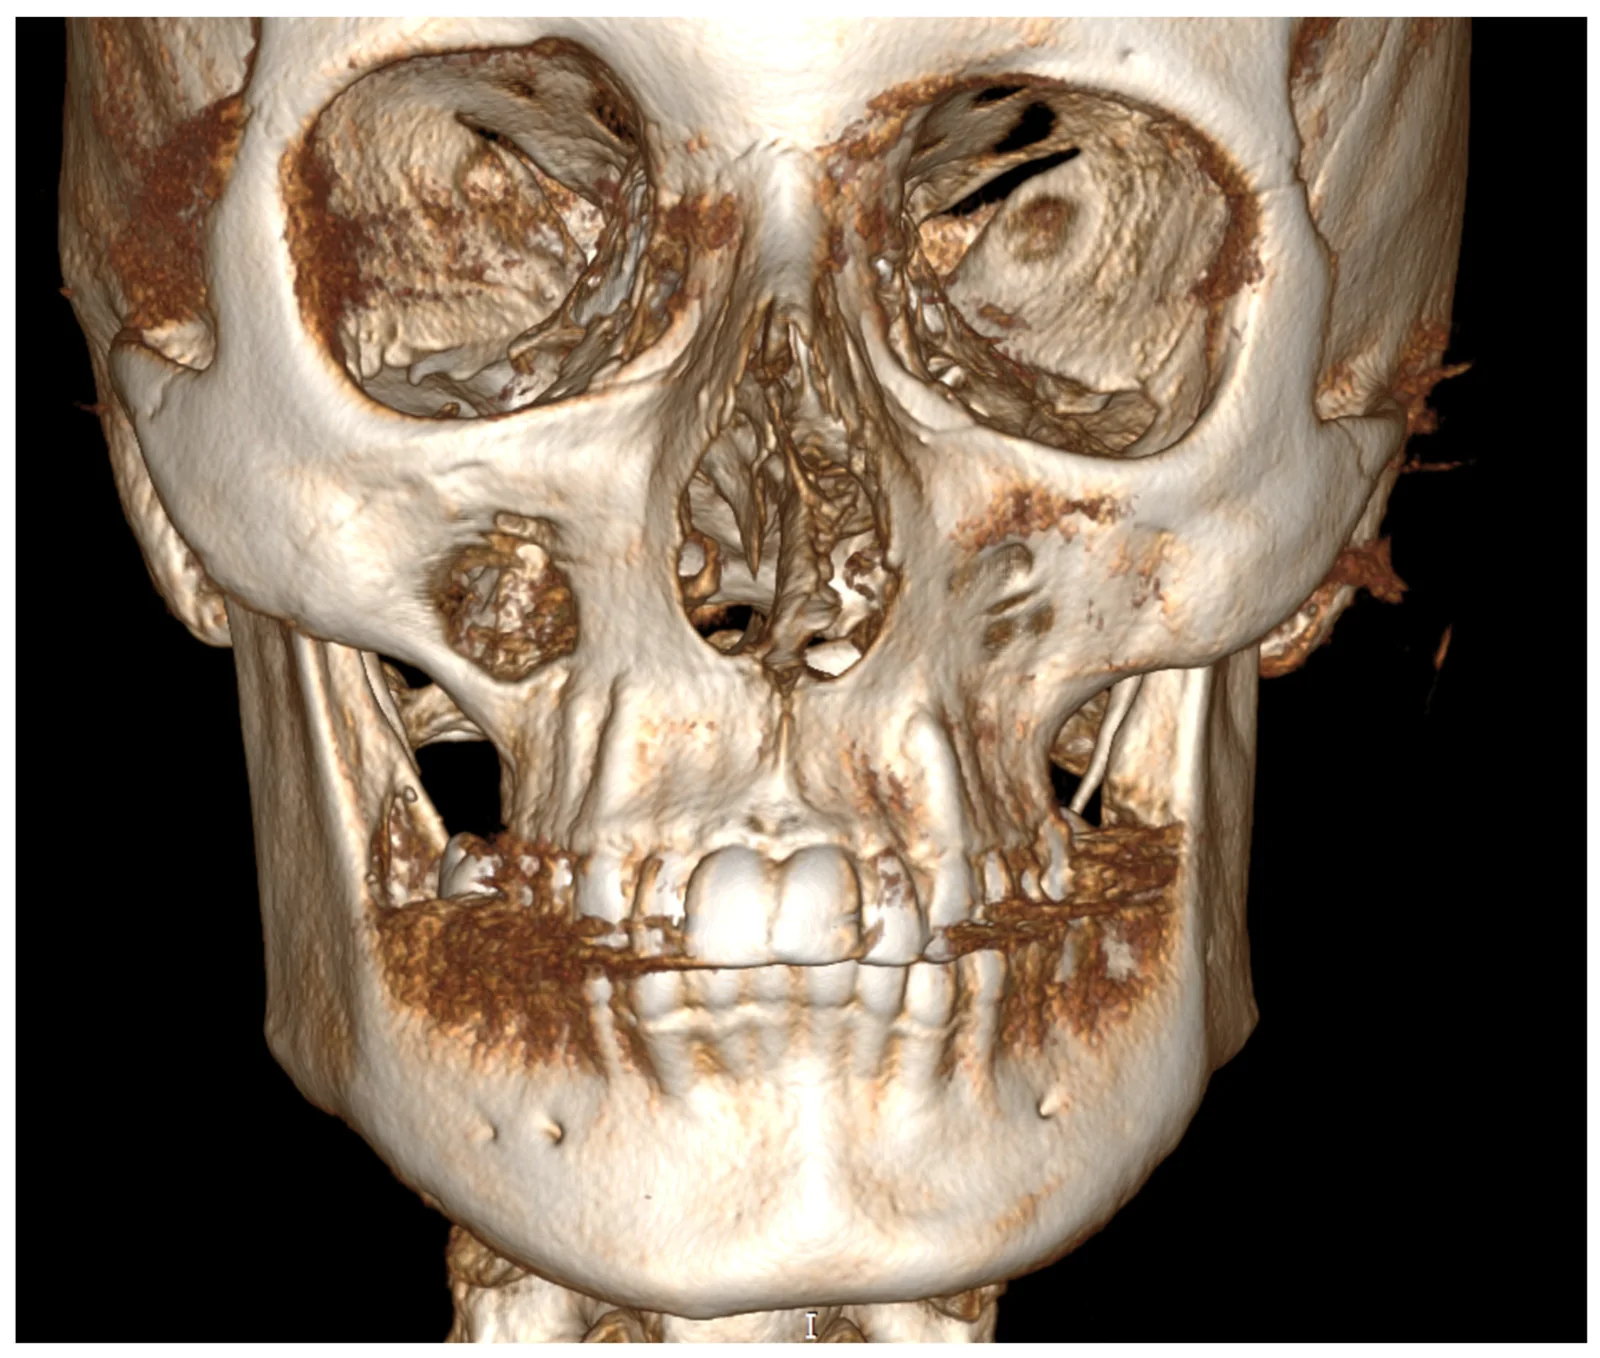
The CLA requires preparation of an antrotomy window whose size and position should be planned according to the extent of access, the periapical pathology, the cyst location, and possible oroantral communication closure. The superior aspect of the approach should take into consideration the infraorbital foramen and its nerve, while the inferior aspect should consider the tooth apices. The 3D-CBCT figure presents the bone loss in the right maxillary sinus wall, due to the CLA, without any significant loss of bone shape, volume, or function. Nevertheless, the CLA is not very common nowadays because of ESS/FESS and other endoscopic approaches. Although it is no longer a routine first-line approach, the Caldwell–Luc procedure may remain a selective surgical option when direct access to the alveolar recess, tooth-bearing structures, or a persistent oroantral defect is required [5,6,7,8,9,10,11,12,17,18,19,20,21,22,28,29,30]. In general, ESS/FESS is preferred when the main problem is osteomeatal complex obstruction or broader sinonasal involvement, whereas a limited Caldwell–Luc approach may be considered for the direct removal of an alveolar recess pathology or foreign bodies and for the simultaneous closure of an oroantral defect; both approaches can be combined when these indications coexist [22,27,28,29,30,33]. A simplified treatment algorithm comparing conservative dental treatment, ESS, the modified Caldwell–Luc approach, and combined approaches is provided in Supplementary Figure S1 [22,27,28,29,30,33]. Perhaps the necessary bone drilling, loss of part of the sinus anterior wall, the down-regulation of maxillary ciliary mucous flow, and sinus ventilation could be some arguments against this approach, yet still, when the source of dental-related infection/inflammation and ODS is caused by still-present dentition and tooth–bone-bearing structures are present, then the CLA should be carefully considered. Some authors emphasize the role of ESS/FESS, others the CLA, while some tend to combine FESS + CLA and perform a dual, two-approach intraoral–intranasal combined treatment, which should also be considered in some special cases. During the patient’s clinical examination, the outcomes just after the procedure and 12 months were stable. Firstly, the considered septoplasty with FESS/ESS was moved to a later stage as a precaution for any ODS relapse or its formation in the opposite left-sided maxillary sinus.
